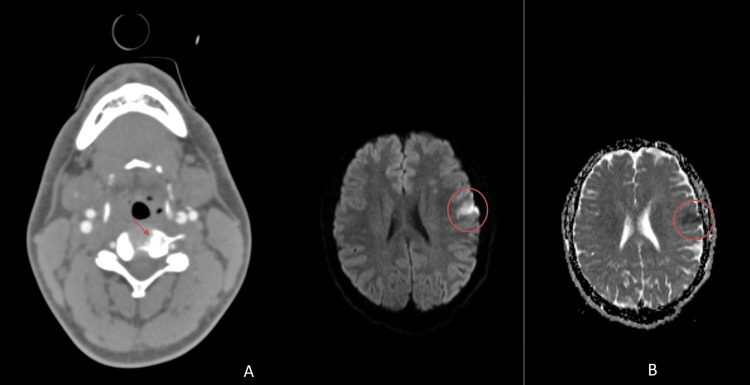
⁠(A) CTA shows a subtle indentation on the medial wall of the initial segment of the left internal carotid artery due to proximity to the left greater horn of the hyoid bone; (B) MRI, DWI shows restricted diffusion in the left posterior frontal area CTA: computerized tomography angiography; DWI: diffusion-weighted imaging; MRI: magnetic resonance imaging
